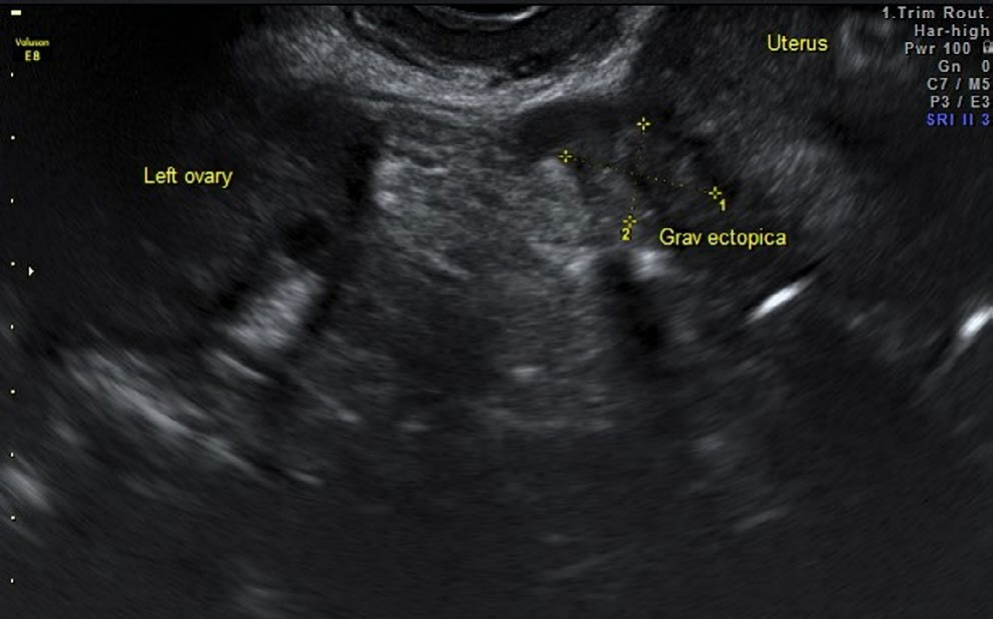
Transvaginal ultrasonography demonstrated a heteroechoic area in the left adnexal view that might correspond to an ectopic pregnancy
